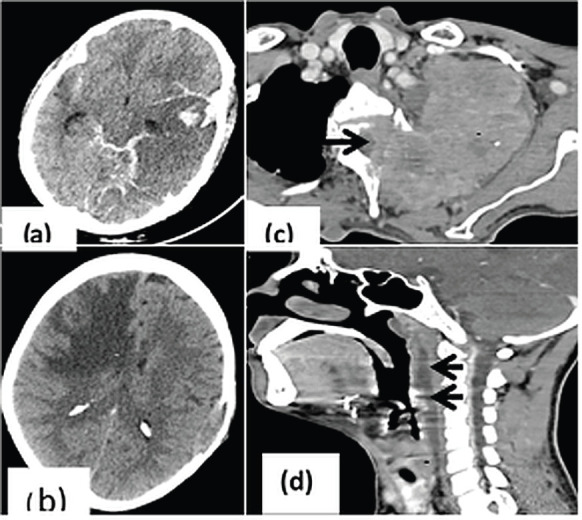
CrC in head and neck region. (a): CT head showing parenchymal as well as subarachnoid bleed in a patient with acute myeloid leukemia; (b): Unenhanced CT showing significant white matter edema (asterisk) in right fronto-parietal region causing mass effect on ipsilateral frontal horn of lateral ventricle; (c): Axial CT image showing pancoast tumour (asterisk) in upper lobe of left lung causing cervical vertebral erosion and intraspinal extension (arrow); (d): Post radiation edema in patient with glottic carcinoma (asterisk) with fluid collection in the retropharyngeal space (arrows) indicating immediate intervention for the same before commencing further treatment.
